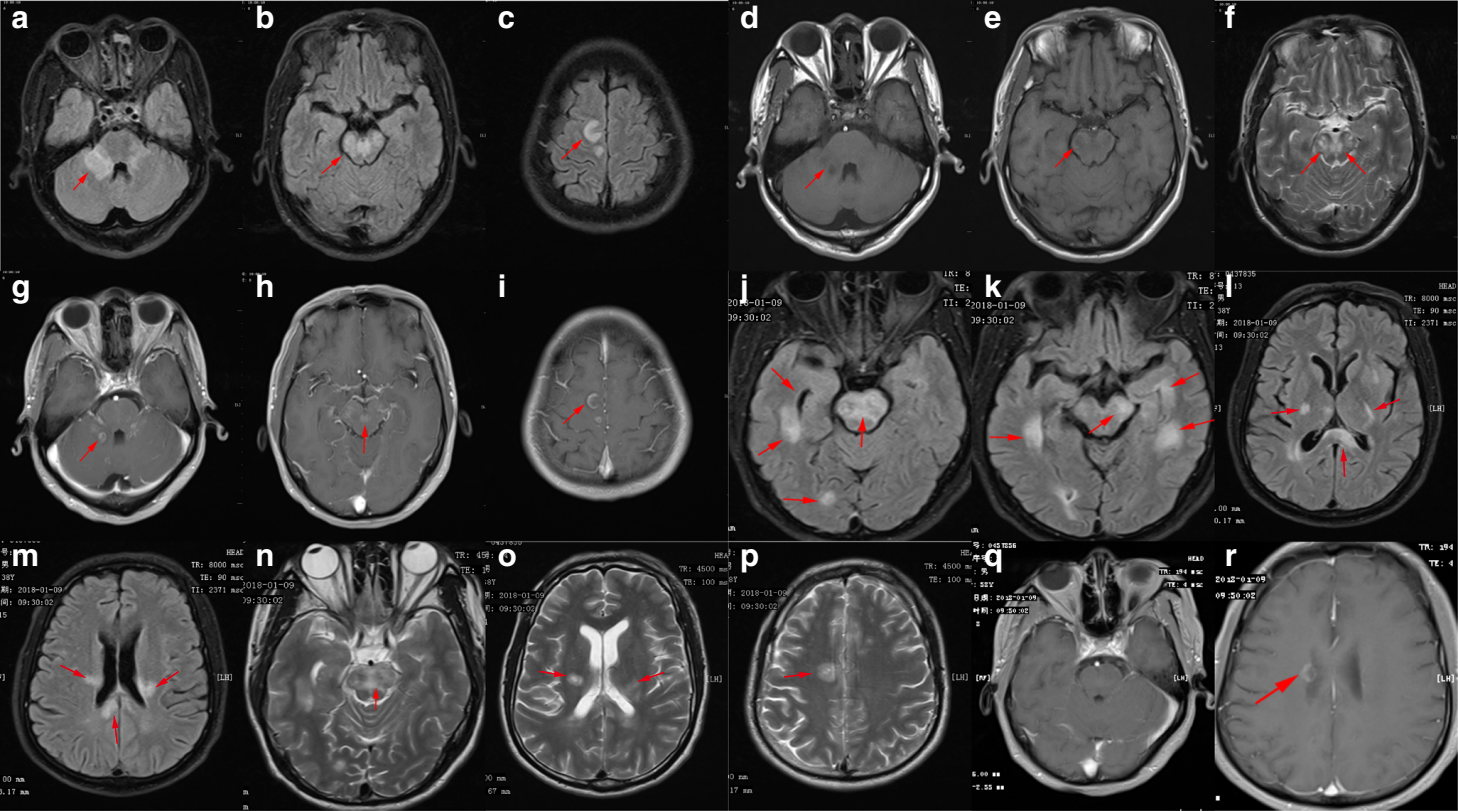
Brain magnetic resonance images of a patient (case 10) with anti-*N*-methyl-d-aspartate receptor encephalitis at first onset (a–i) and relapse (over 2 years after first onset) (j–r). Fluid attenuation inversion recovery (FLAIR) sequence showing hyperintense lesions bilaterally in the medi-peduncle (a, arrow), most of the midbrain (b, arrow), and the local parietal lobe (c, arrow). T1-weighted sequence showing low signal intensity in the aforementioned lesions (d, e, arrow). T2-weighted sequence showing multiple different-sized hyperintense lesions (f, arrows). Contrast-enhanced FLAIR sequence showing ring-shaped (g, arrow), irregularly shaped (h, arrow), and C-shaped (i, arrow) lesions in the medi-peduncle, midbrain, and parietal lobe, respectively. FLAIR sequence showing hyperintense lesions in the whole pons (j, arrow), occipital lobe (j, arrow), whole midbrain (k, arrow), left hippocampus (k, arrow), bilateral temporal lobes (k, arrow), basal ganglia (l, arrow), splenium of the corpus callosum (l, arrow), and paraventricular regions bilaterally (m, arrow). T2-weighted sequence showing irregularly shaped, multiple, different-sized, and circular hyperintense lesions in the pons (n, arrow), bilaterally in the basal ganglia (o, arrow), and parietal cortex (p, arrow), respectively. Lesions are not visible in the pons (q), but the C-shaped lesion (r, arrow) is visible in the paraventricular region in the contrast-enhanced sequence
